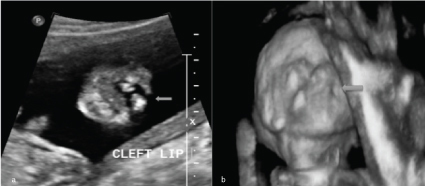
(a) Two-dimensional sonography depicting left paramedian cleft lip (arrow). (b) Cleft lip on three-dimensional sonography (arrow).
